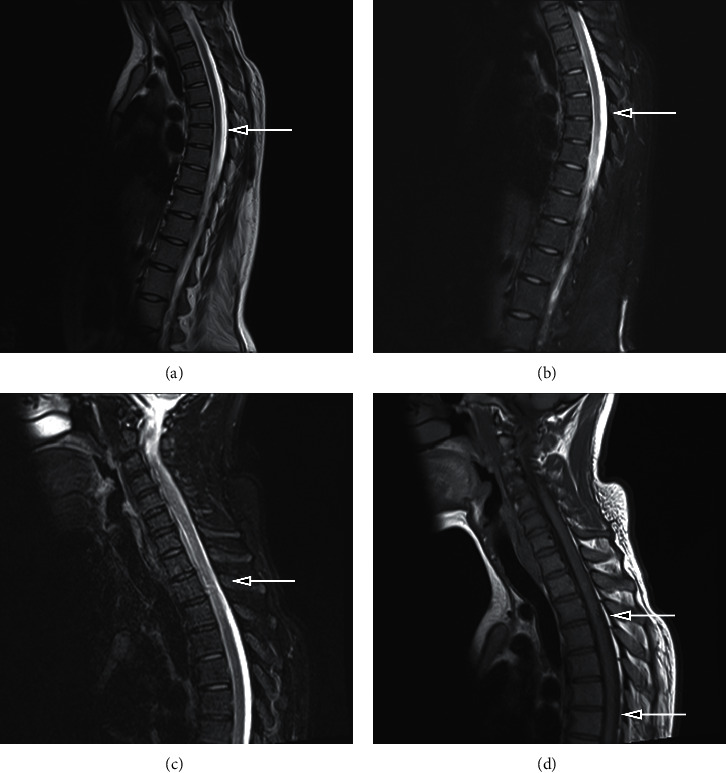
Magnetic resonance imaging (MRI) of the thoracic spinal cord. The gray arrow indicates the site of the thoracic spinal cord was smaller than the healthy people.
